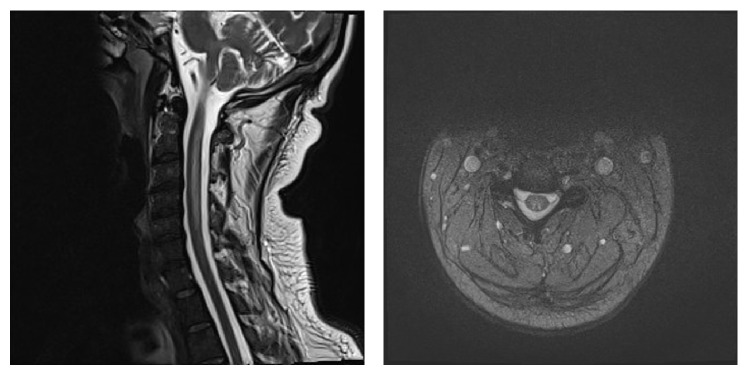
T2 weighted MRI in both axial and sagittal views of the spine shows long segment of abnormal high signal involving the posterior aspect of the cervical cord extending from C1 to C5.
